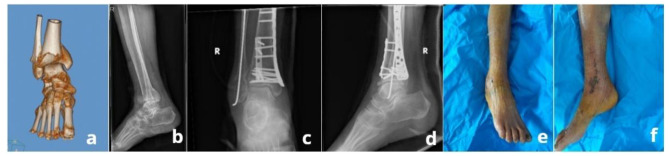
Photographs and radiographs from an 82-year-old female who experienced a varus-type pilon fracture (AO/OTA type C3). (a ~ b) Preoperative radiographs showed the fracture with a large anterior fragment involving the lateral column. (c ~ d) A 1/3 tubular plate was used to stabilize the anterior and posterior fragments, with the fibular fracture fixed intramedullary with a Kirschner wire. (e ~ f) The patient presented with local edge necrosis in the medial wound and the wound healed 12 days beyond the average healing time after dressing changes
